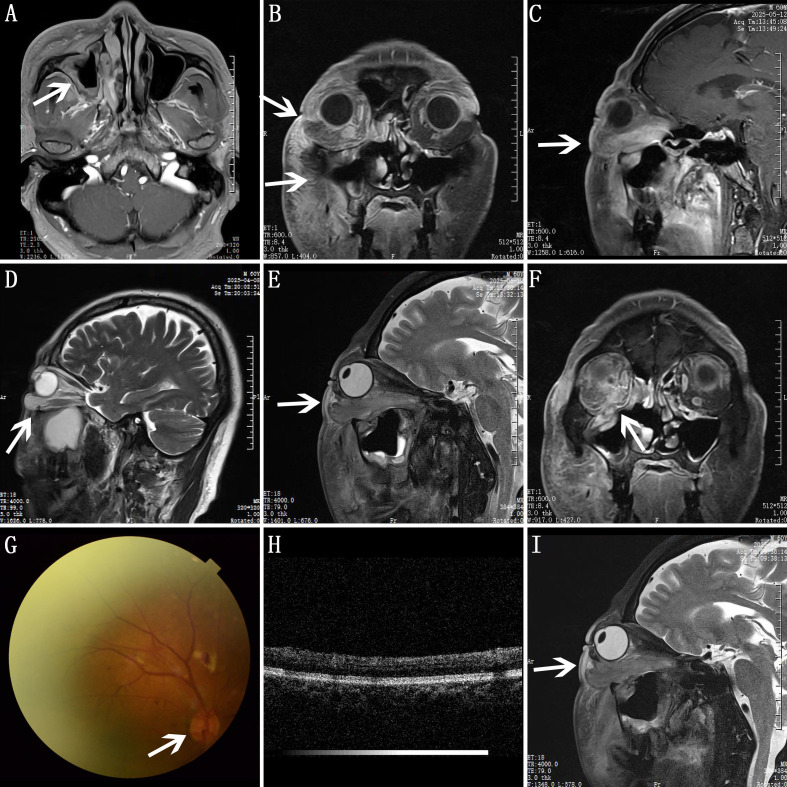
(A–C) MRI reveals that there was increased signal in the fat space behind the lower part of the right eye, accompanied by swelling of the right inferior rectus muscle. Right-sided proptosis was present, suggesting an inflammatory lesion. (A) Normal changes during postoperative recovery. (B) The coronal view. (C) The sagittal view. (D) The inferior rectus muscle region of orbital MRI before the FESS surgery. (E) The inferior rectus muscle region of orbital MRI after the FESS surgery. (F) The enhanced MRI showed bone defects. (G) Fundus photography of the right eye during the patient’s second hospitalization after blindness. (H) Optical coherence tomography of the right eye during the patient’s second hospitalization after blindness. (I) The inferior rectus region of orbital MRI after blindness.
